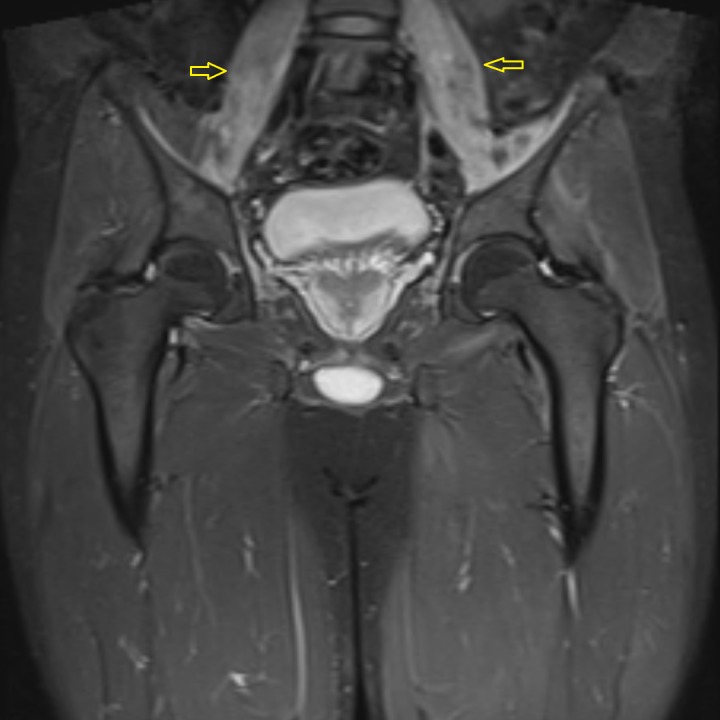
MRI pelvis (coronal view) Coronal fat-saturated, fluid-sensitive sequence without contrast showing hyperintensity in the bilateral psoas and iliacus muscles (arrows) suggestive of myositis.
